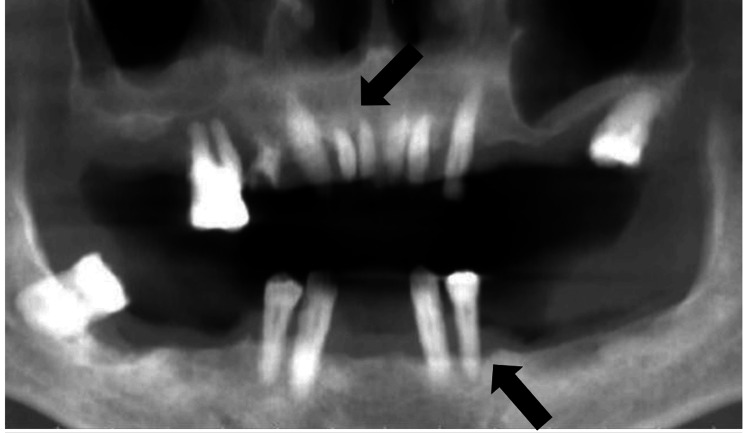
Preoperative cone-beam computed tomography image. The arrows indicate bone loss in both the maxilla and mandible.
